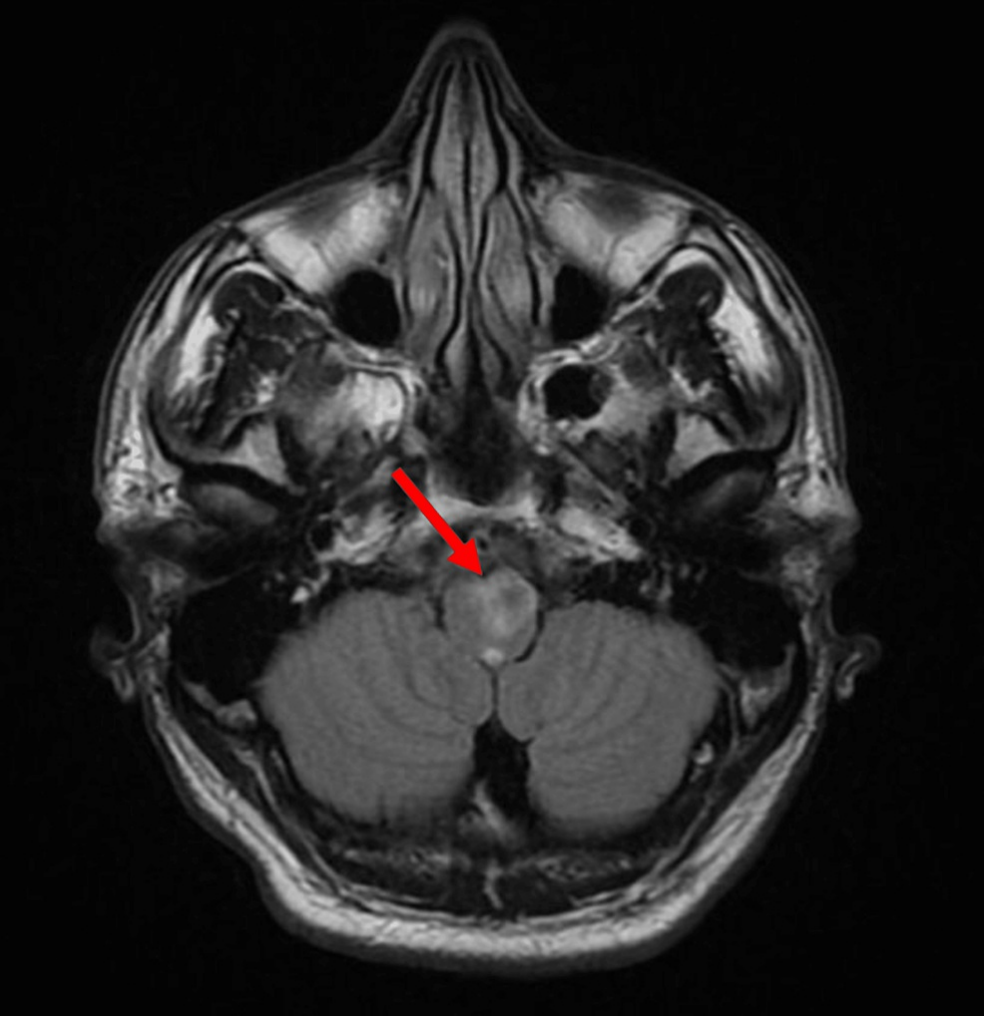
Axial T2 fluid-attenuated inversion recovery (FLAIR) demonstrating a new ring-enhancing lesion at the left medulla oblongata (arrow) associated with a halo of vasogenic edema​.
